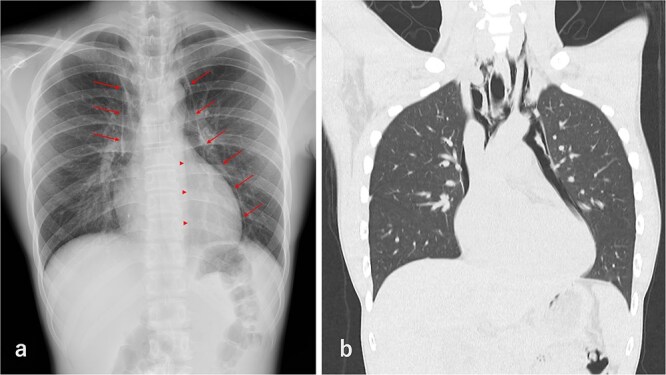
(a) Gas collection between the visceral and parietal pleura (arrows) and a lucent area along the left border of the descending aorta (arrow heads). (b) Sagittal plain chest computed tomography image.
